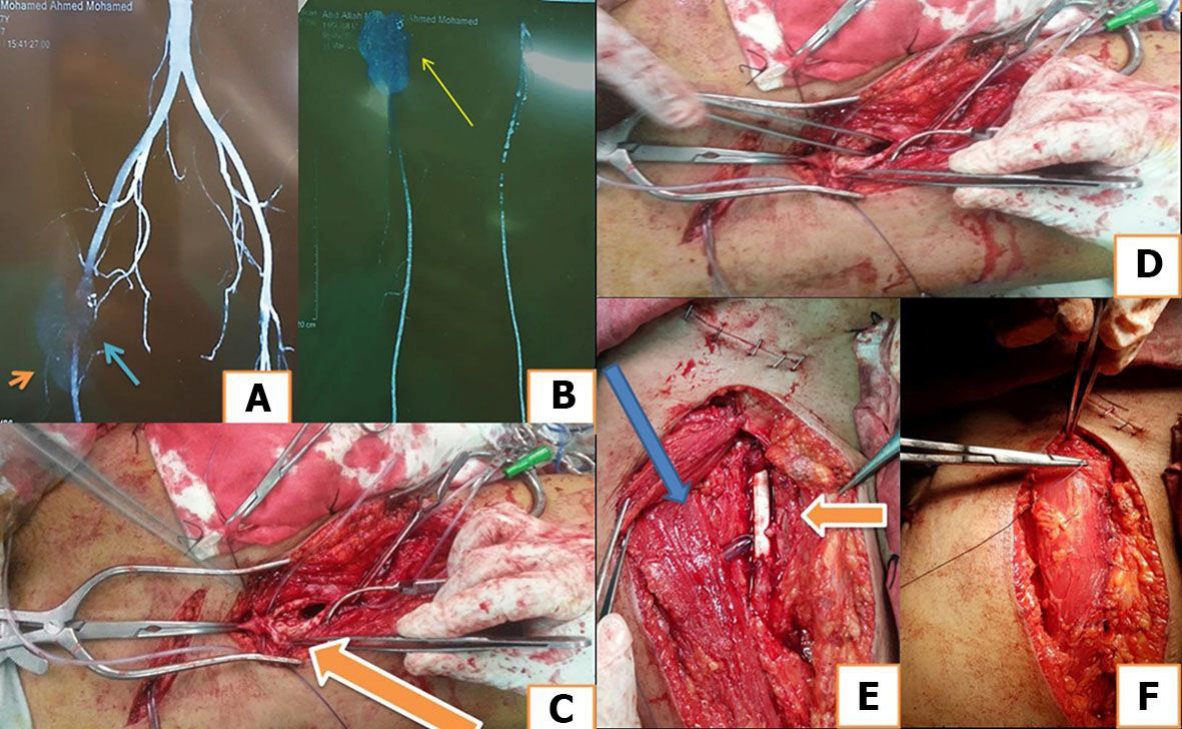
(A) Blue arrow indicates Behçet's pseudoaneurysm of the right common femoral artery involving the origin of the profunda femoris artery (orange arrow), seen on computed tomography angiography (CTA); (B) yellow arrow indicates size of the pseudo aneurysm (5x5cm); (C, D) orange arrow showing pseudoaneurysm opened after proximal and distal control, (E) orange arrow showing proximal and distal anastomoses with 6x60 cm PTFE graft and ligation of distal profunda femoris artery with Sartorius muscle flap (blue arrow); (F) showing closure of the wound after Sartorius muscle flap from same side.
